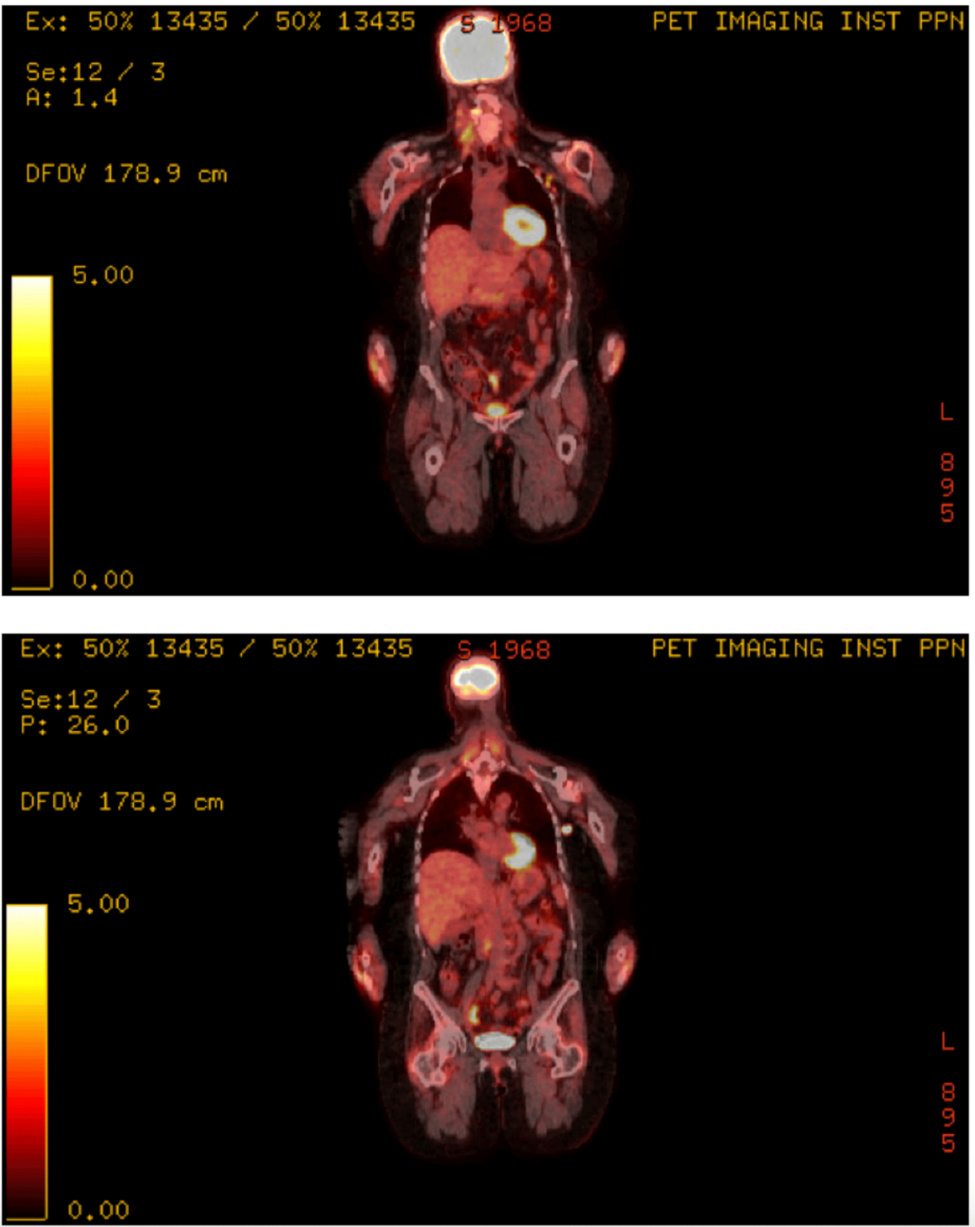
Sequential PET-CT scans. Intense hypermetabolic activity in cervical and left axillary lymph nodes, initially suggestive of relapse.
